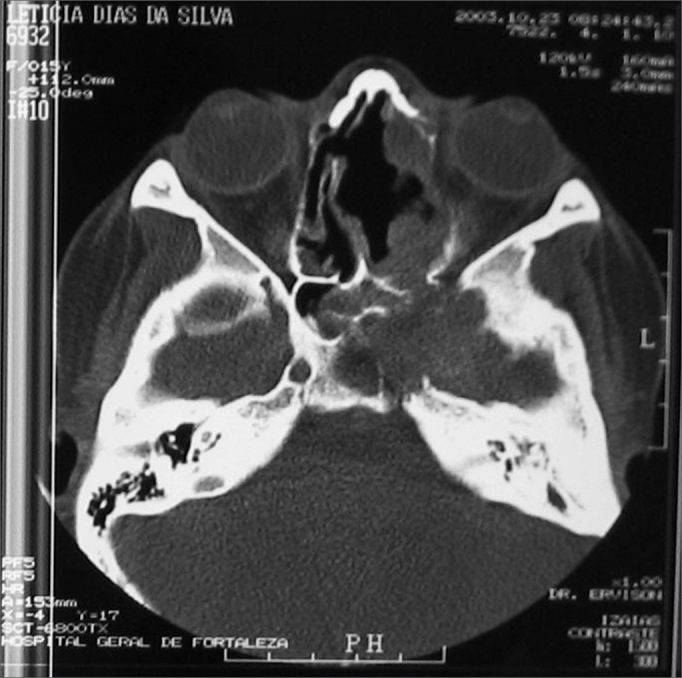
CT scan - axial view of a relapsed nasosinusal hemangiopericytoma (case 4).
